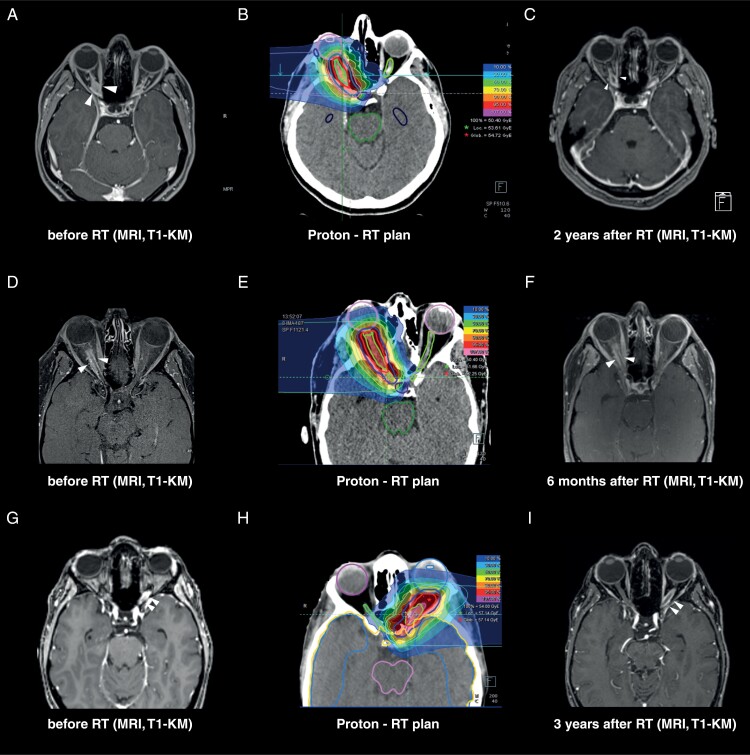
Treatment response following proton beam radiotherapy. Exemplary case with optic nerve sheath meningioma (ONSM)-03: (A) Right-sided ONSM (white arrows) on T1-weighted contrast-enhanced MRI before the start of proton radiotherapy. (B) Proton radiotherapy treatment plan with a total dose prescription of 50.4 Gy (RBE) in 1.8 Gy (RBE) single dose (100%), and colored isodose lines (in %). Proton radiotherapy was administered using a lateral beam arrangement to minimize radiation dose in the adjacent organs at risk (eg, lens, brain). In the specified beam direction, negligible radiation dose is deposited distal to the target volume or Bragg peak, eg, in the contralateral eye as preservation of contralateral visual outcome is crucial. (C) Increasing central T1-hypointensity within the ONSM as a sign of treatment response after 2 years following proton radiotherapy. Additional exemplary proton radiotherapy plans with corresponding MRI prior and following Radiation therapy (RT) (ONSM-04: D-F, ONSM-08: G-I).
